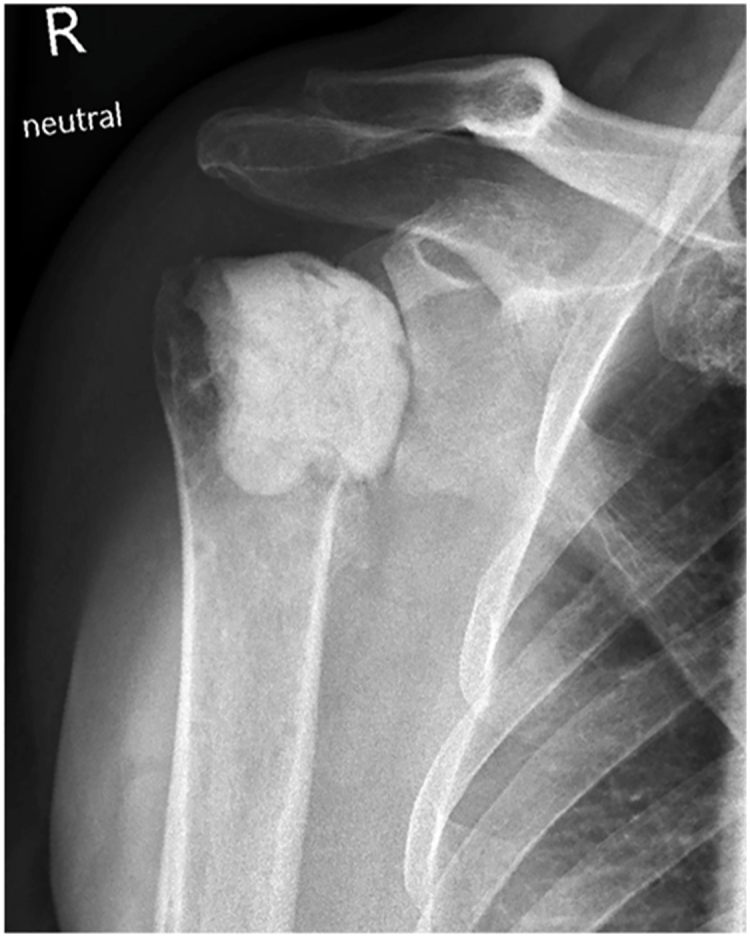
Anteroposterior radiograph of the right shoulder showing a cement spacer after the first revision surgery.
